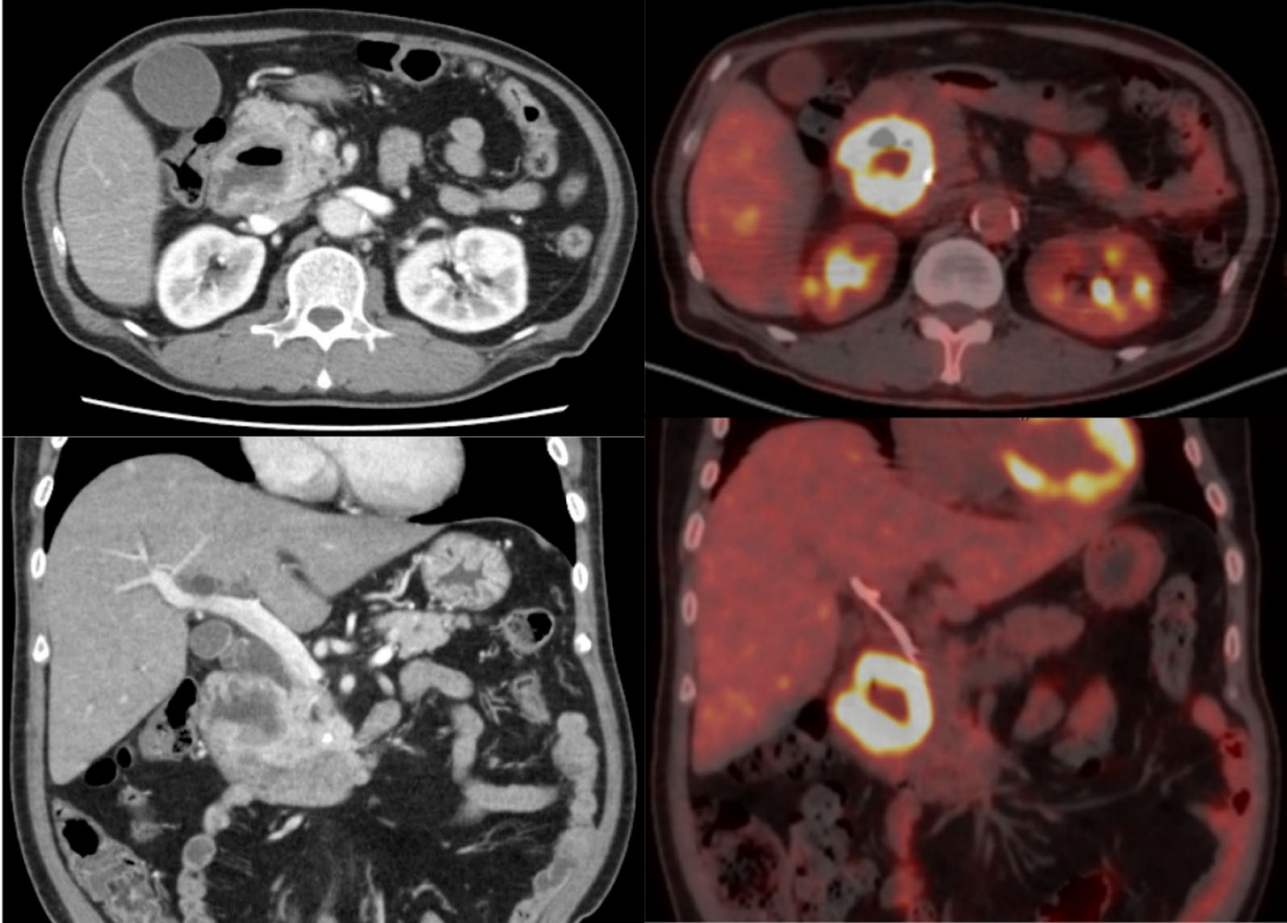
Findings of contrast-computed tomography (CT) and positron emission tomography (PET)–CT: contrast-CT revealed an ill-defined lesion involving the second portion of the duodenum, predominantly along its medial wall, measuring a diameter of 60 mm. Bulging growth into the duodenal lumen and the pancreatic head, suggesting infiltration of the lesion, was observed. In addition, associated with gallbladder enlargement, bile duct dilation due to the stenosis of the lower bile duct, and dilation of the main pancreatic duct. No metastasis was observed on PET–CT
